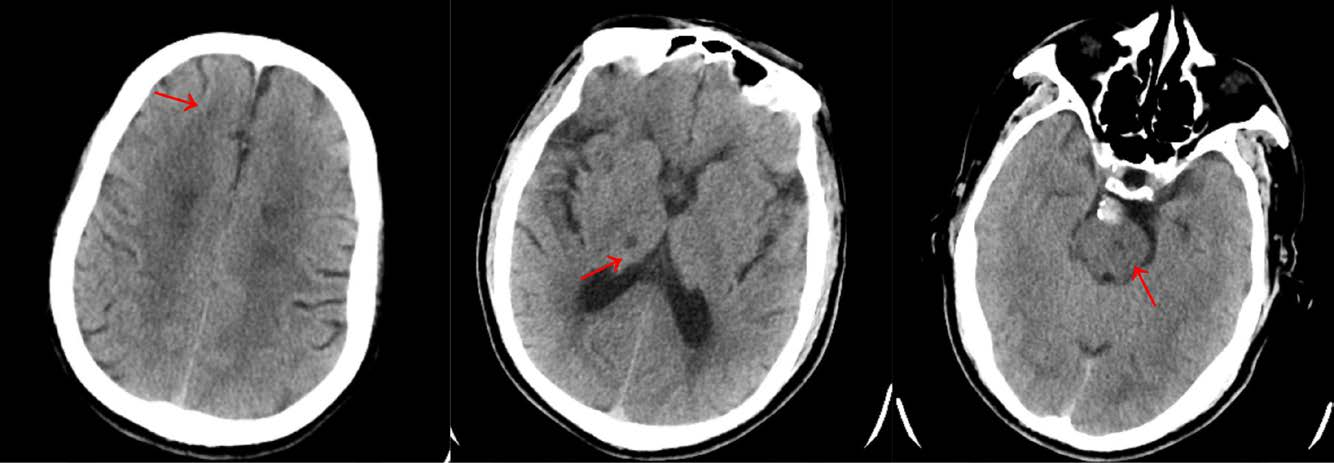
Cranial CT showing multiple lacunar infarctions in the bilateral frontal and parietal lobes, basal ganglia, thalamus, and brainstem. CT = computed tomography.
